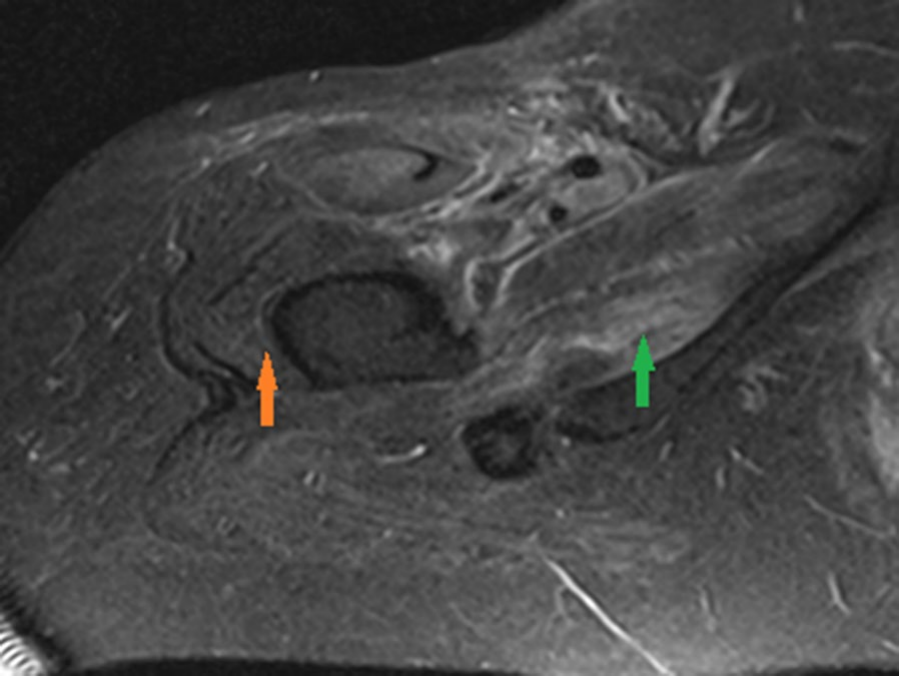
Magnetic resonance imaging (MRI) of the right femur in axial inversion recovery sequence image demonstrates extensive signal abnormality within the right hip muscles which shows features of muscle edema (green arrow). The orange arrow demonstrates the normal muscle
